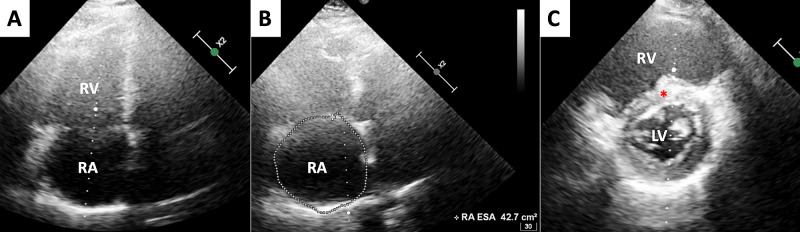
Transthoracic echocardiography: Functional and morphological evaluation (A,B) Four Chambers view, end-diastolic phase. The images show dilated right atrium (42.7 cm^2^ area) and right ventricle (RV). (C) Short axis view. The image confirms RV dilation with a mild interventricular septal flattening (red asterisk) and a D-shaped appearance of the left ventricle.
